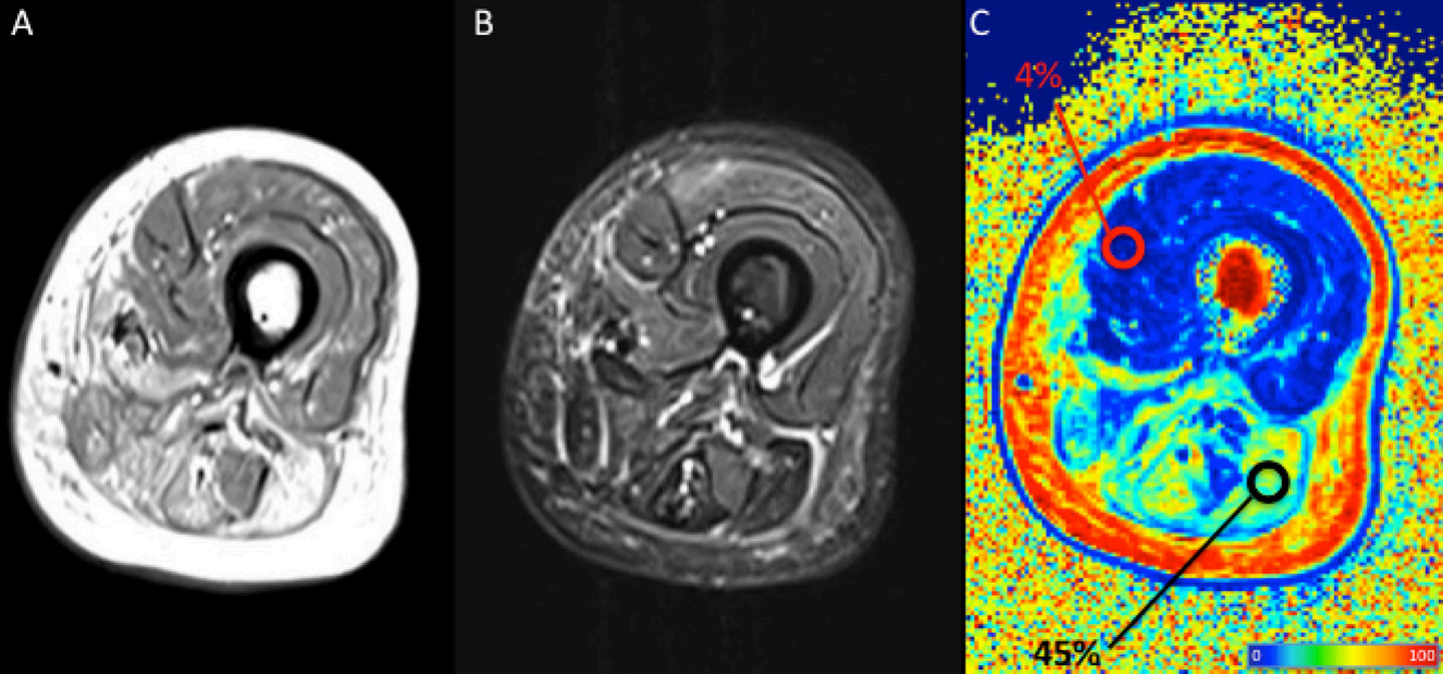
Qualitative and quantitative left thigh muscle MRI in an adult subject with facio-scapulo-humeral disease (FSHD). (A) T1-weighted, (B) short-tau inversion recovery (STIR), and (C) fat fraction (FF) calculated with a 3-point Dixon approach are displayed. Regions-of-interest (ROI) are positioned, respectively on normal and fatty replaced muscles, with their mean FF shown as percentage in the tag. The figure shows extensive fatty replacement of the harmstring muscles (mean FF = 4%), with the ROI positioned on the long head of the biceps femoris, compared to the normal quadriceps (mean FF = 45%). Colors from dark blue to dark red represent the percentage of fat (FF) (see color bar). MRI images have been collected during routine patient care.
